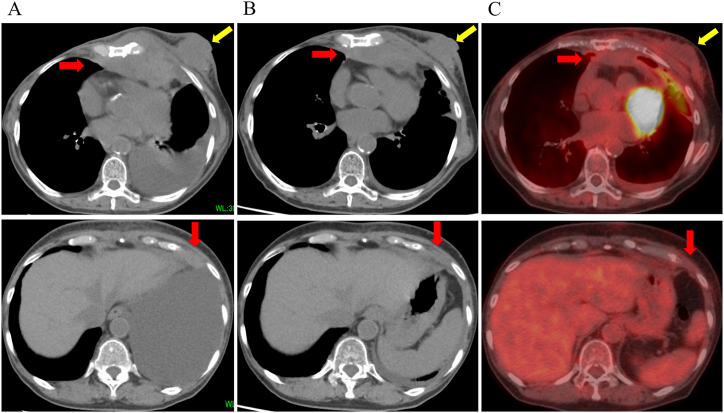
Temporal changes in tumor lesions on CT images. (A) A growing thymic carcinoma in the anterior mediastinum (red arrow), a disseminated lesion of thymic carcinoma in the cardiac diaphragm angle (red arrow) and a growing breast carcinoma in the left breast (yellow arrow) are shown. (B) Two months after irradiation and pembrolizumab administration, the lesions tended to shrink, and the disseminated lesion, which was outside of the irradiation range, also showed shrinkage. (C) Four months after the start of treatment, the lesions in the anterior longitudinal cage and left breast and the disseminated lesions were further reduced. CT, computed tomograph.
